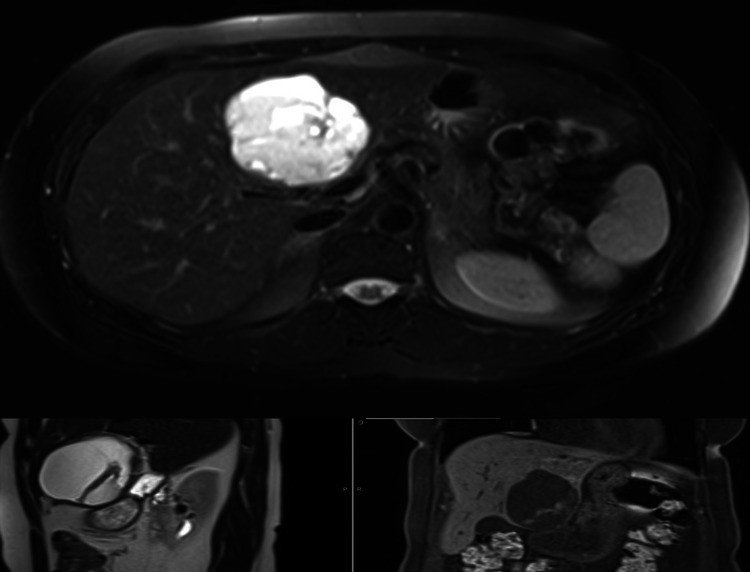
MRI of the liver shows a complex cystic mass lesion in the left lobe of the liver
